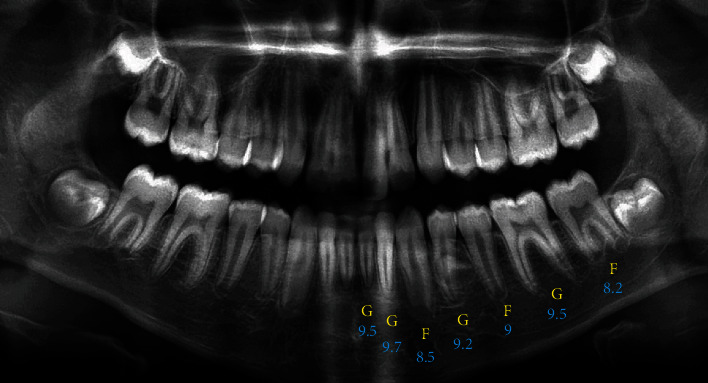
The scoring of the teeth on a panoramic radiograph according to the Demirjian (yellow), Nolla (blue), and Willems (yellow) methods.
